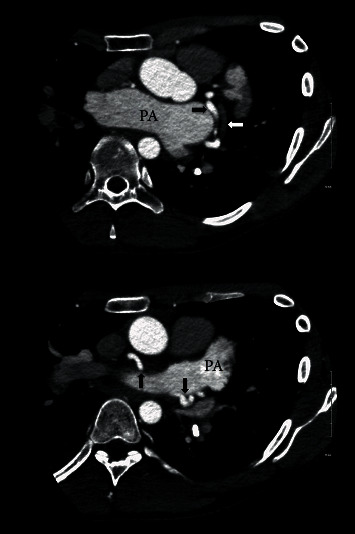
Thoracic computed tomography showing course of fistula (black arrows) originating in the left main coronary artery, initially running parallel to left circumflex artery (white arrow), posterior, and above the pulmonary artery (PA) before draining into the right atrium (right atrium not visualized in these sections).
